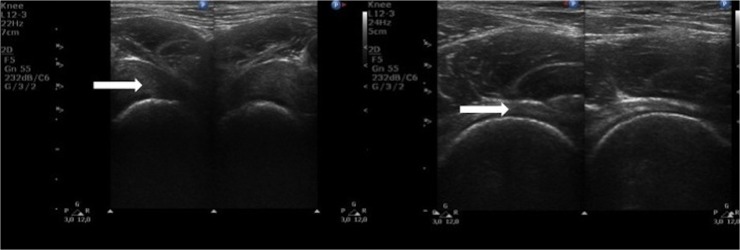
Ultrasound images of hip joints. (Left) Transverse scan of hip joint a 14-year-old patient with a severe phenotype of MPS II. (Right) Longitudinal scan of hip joint in a 14-year-old healthy child. Arrows shows differences in the distance from the femoral neck to joint capsule (synovial joint space, SJS).
